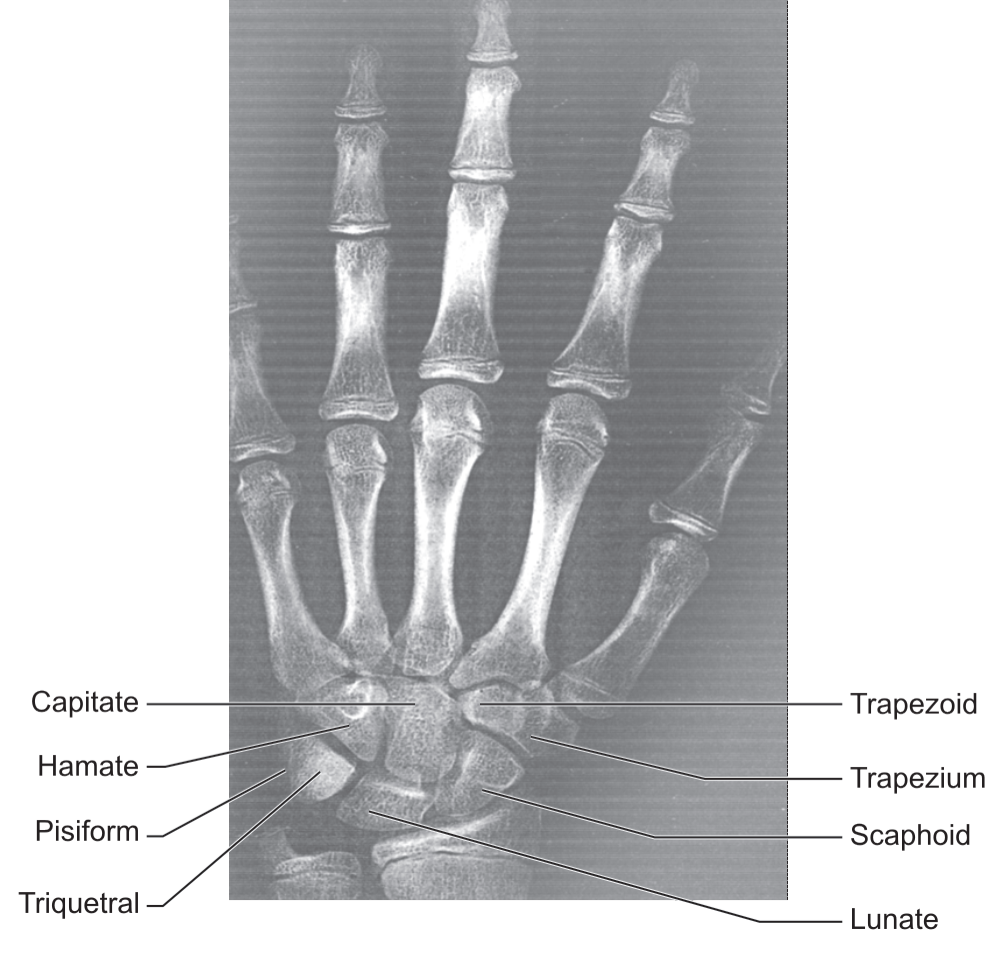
Hand and wrist radiograph showing carpal bones
